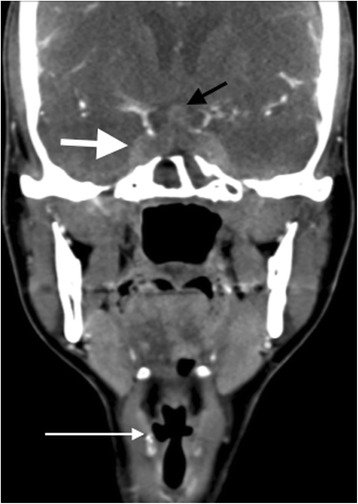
Coronal contrast-enhanced CT image of the skull base and neck (pre-cyclophosphamide treatment). There is marked abnormal chronic inflammatory infiltration of the cavernous sinuses bilaterally (bold white arrow) and hypophyseal involvement (black arrow). Multiple lower cranial nerve palsies (Collet-Sicard syndrome) as a result of the skull base inflammatory involvement were evident on imaging, including right vocal cord palsy indicated by adducted right true vocal cord and enlarged right laryngeal ventricle (long white arrow)
